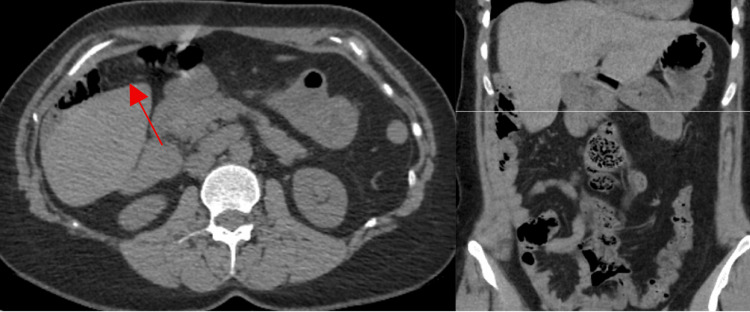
Selected coronal section and corresponding axial section of abdominal and pelvic CT without contrast Postoperative review of the previous scan identified the corresponding site of omental adhesion (red arrow). CT: computed tomography
